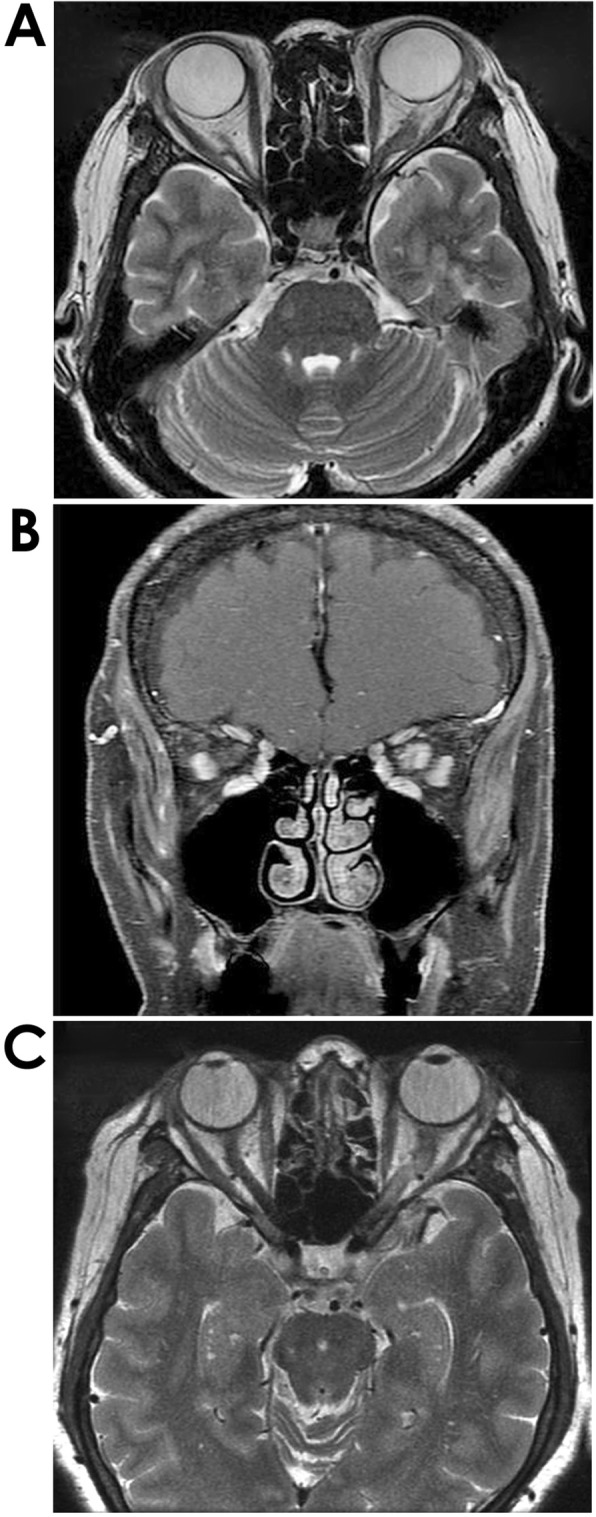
Brain magnetic resonance imaging (MRI). Seen at the initial visit, the axial section revealed irregular hypertrophy of the left retrobulbar optic nerve (a). Moreover, the post-contrast T1-weighted image showed enhancement of the left retrobulbar optic nerve (b). Five months later, a T2-weighted image indicated that the hypertrophy at the left retrobulbar optic nerve was resolved (c)
